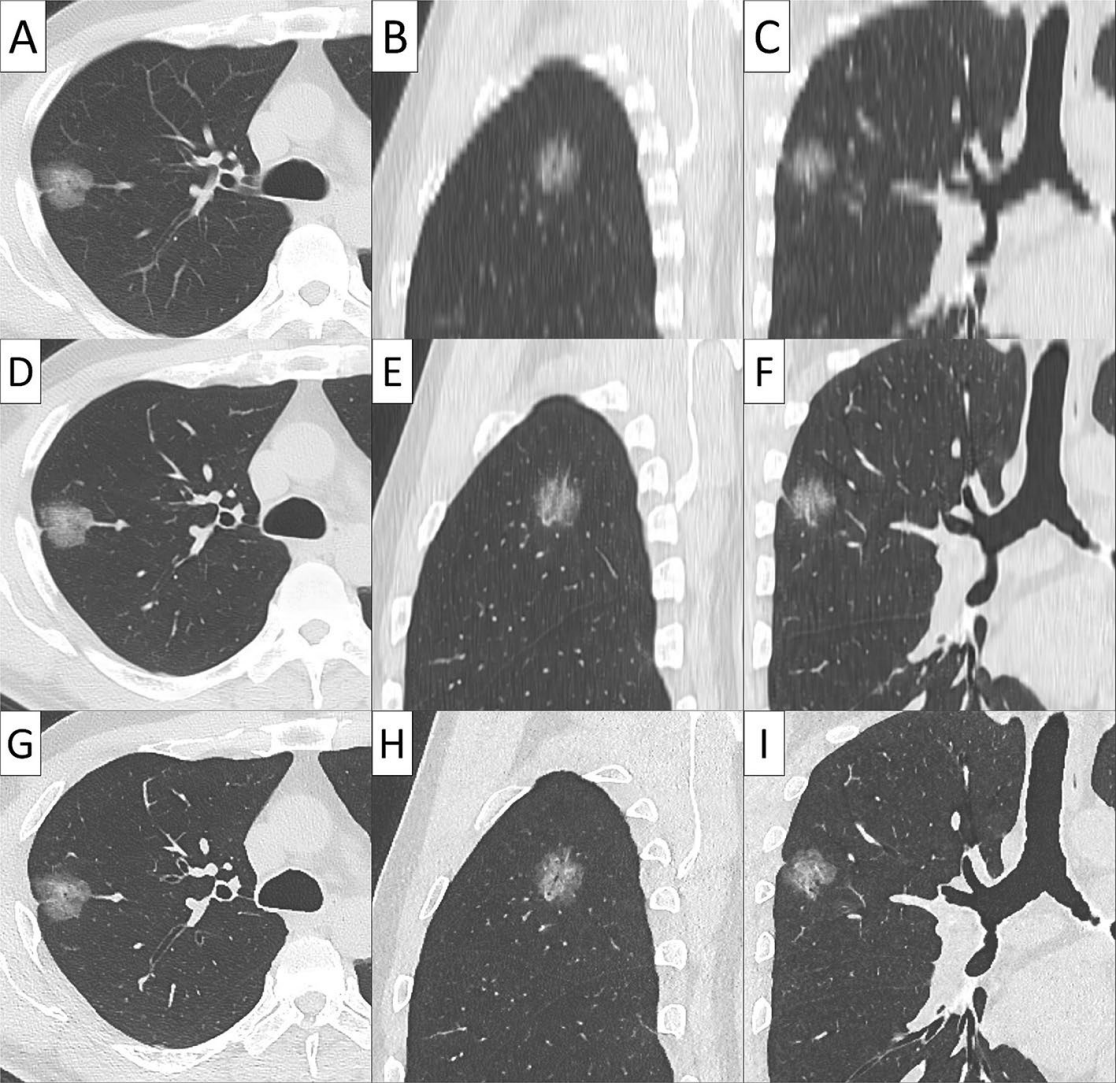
Papillary adenocarcinoma in a 47-year-old male. (A) Axial view, (B) sagittal view, and (C) coronal view of conventional 5-mm thick CT. (D) Axial view, (E) sagittal view, and (F) coronal view of virtual thin-section CT (TSCT). (G) Axial view, (H) sagittal view, and (I) coronal view of real TSCT.
